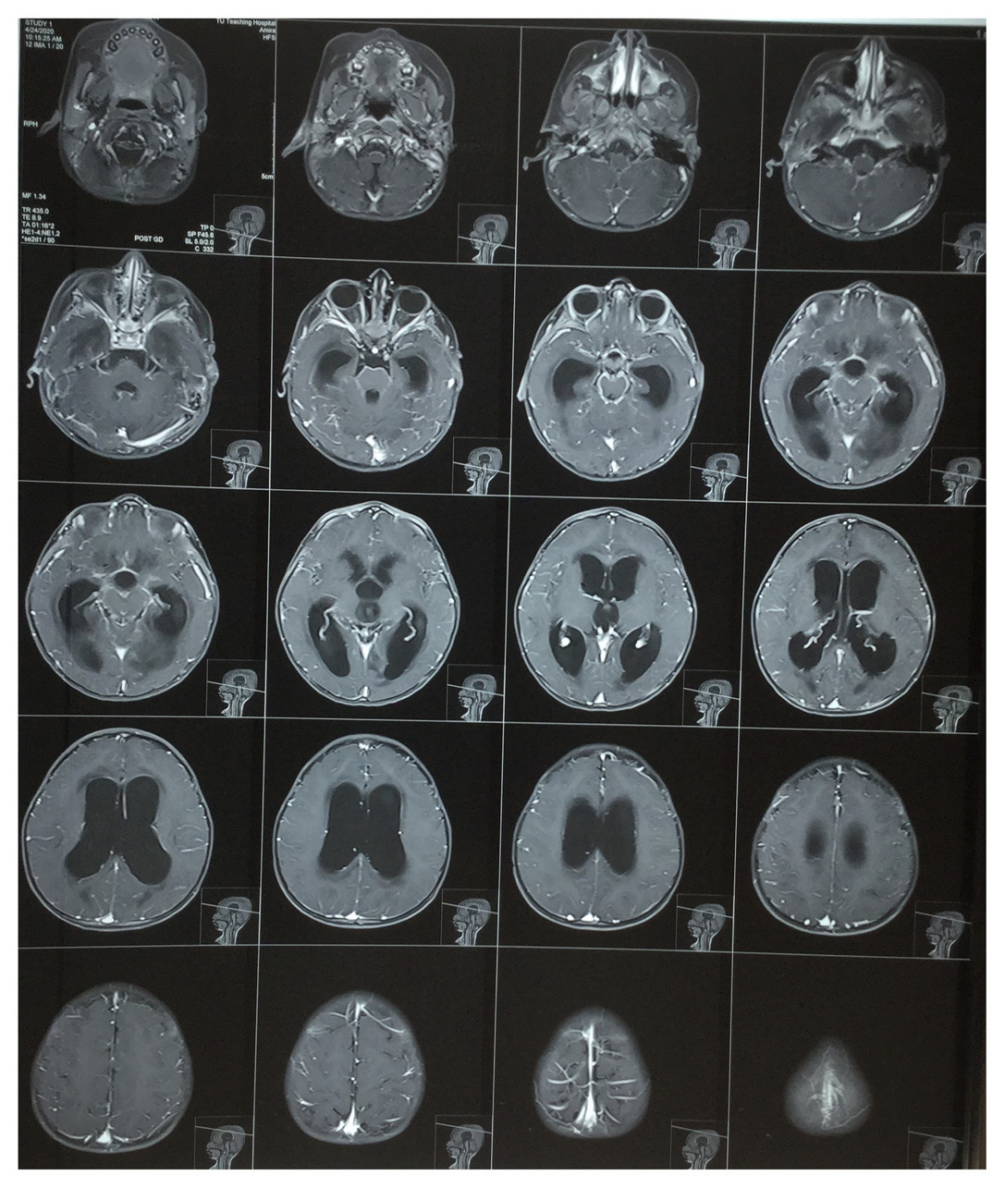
Contrast-enhanced magnetic resonance imaging (MRI) of the brain also showed features of noncommunicating hydrocephalus. Leptomeningeal enhancement was seen in the bilateral temporal regions, suggestive of leptomeningitis with aqueduct obstruction with exudates due to meningitis.
